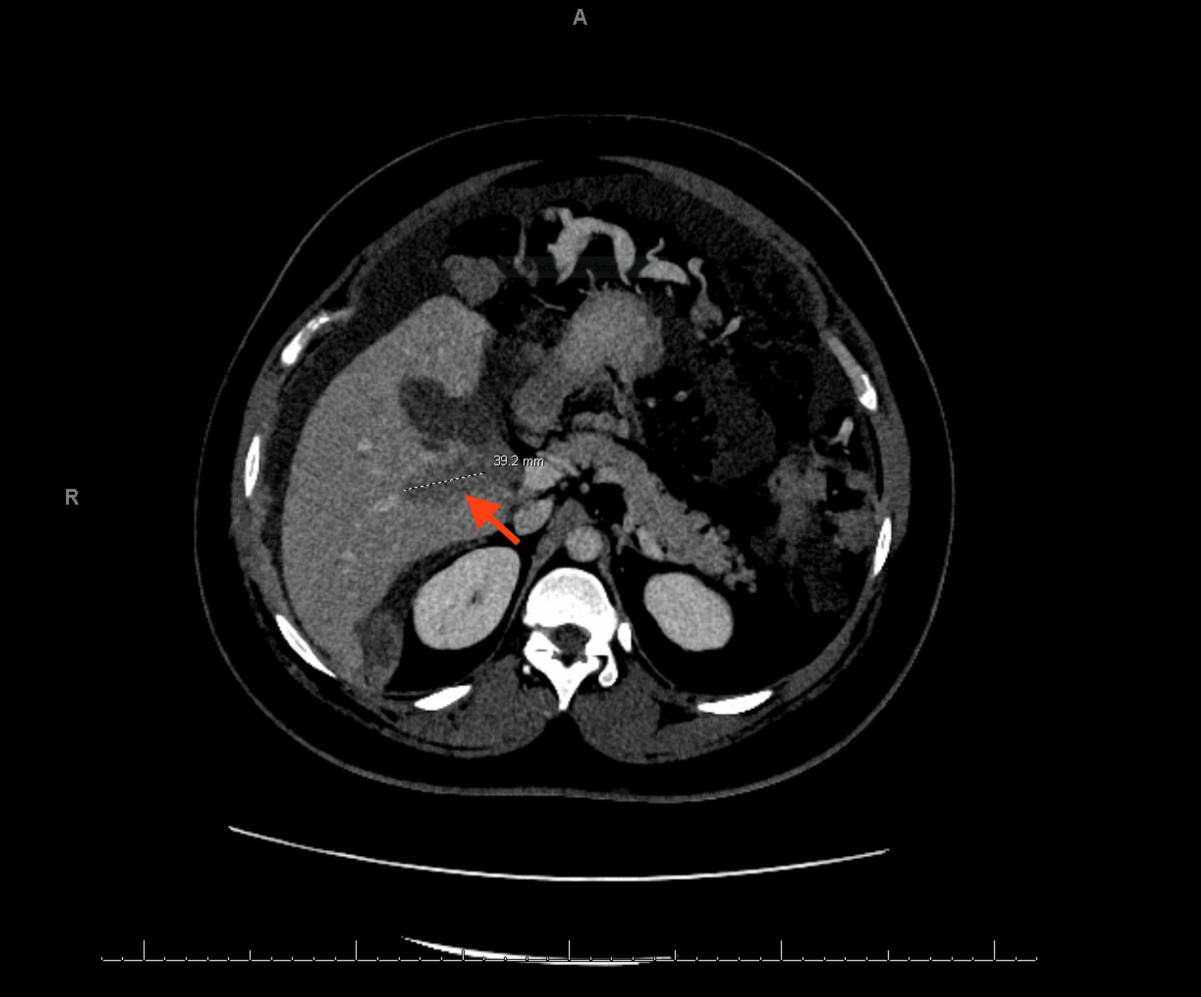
CT scan showing a 40 mm liver lesion. The red arrow indicates the metastatic spread of the tumor to the liver.
